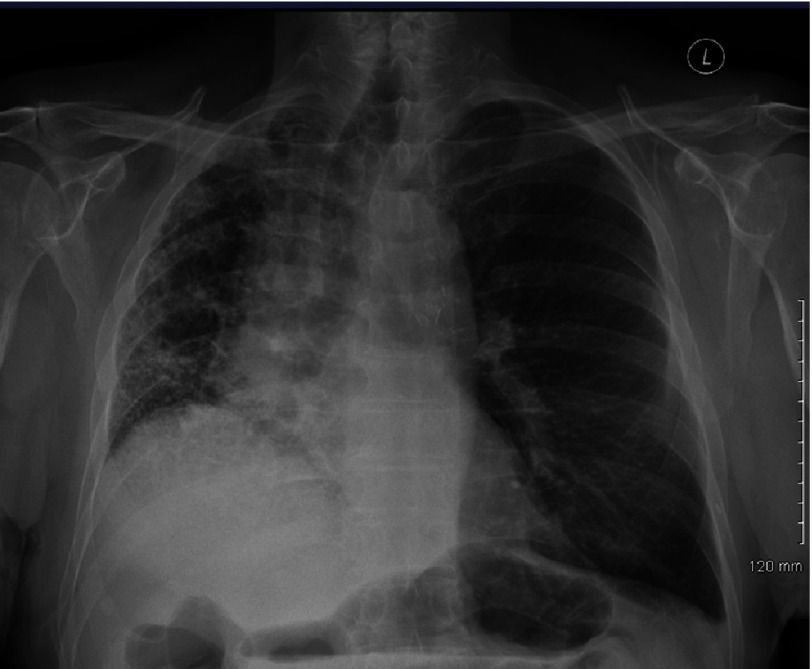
Chest radiograph of a 71 year old recipient 5 months after left single lung transplant for IPF. The allograft is well expanded with mediastinal shift towards the native lung. The forced vital capacity was 72% of predicted and FEV_1_ was 87%.
